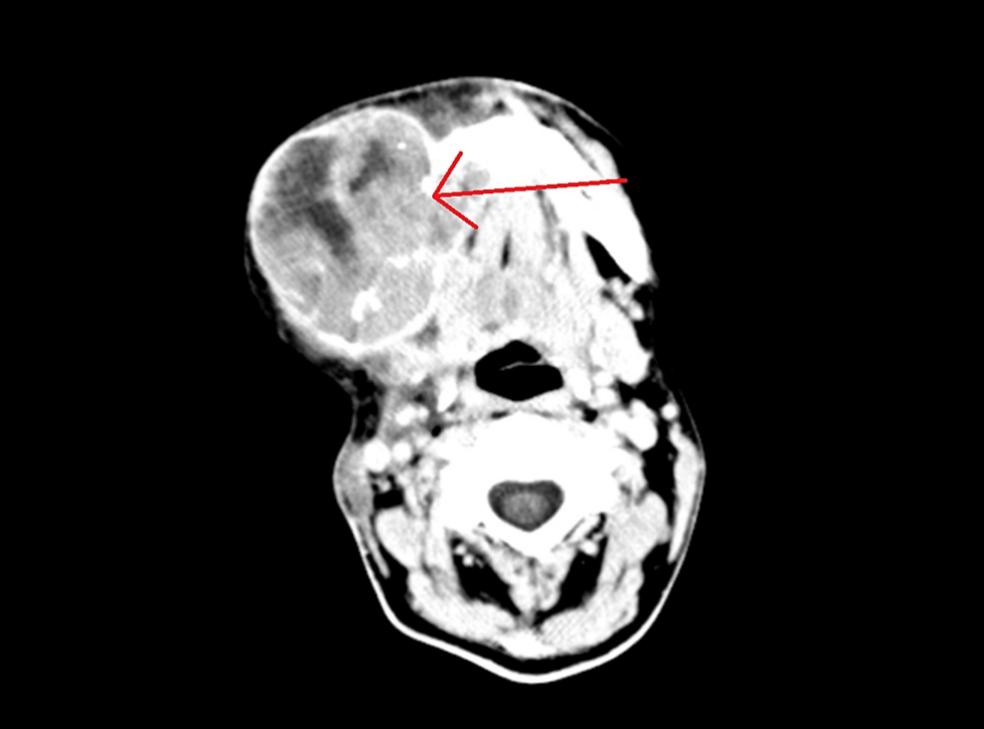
Cerebral MRI axial view. Magnetic resonance imaging (MRI) representation of the right mandibular deep infiltrative tumor recurrence measuring 93 mm anteroposterior diameter, 55 mm laterolateral diameter, and 100 mm craniocaudal diameter, exhibiting inhomogeneous gadolinophilia and central necrosis. The tumor is invasive, extending medially into the oral floor adjacent to the pharyngeal Rossenmuller recess, posteriorly reaching the carotid lodge and causing displacement, and cranially contacting the lateral wall of the right maxillary sinus (zygomatic complex). Anteriorly, it extends to the mandibular chin, enveloping the mandibular lateral incisors. Additionally, it invades the masseter and right pterygoid muscles, as well as the superficial subcutaneous tissue.
